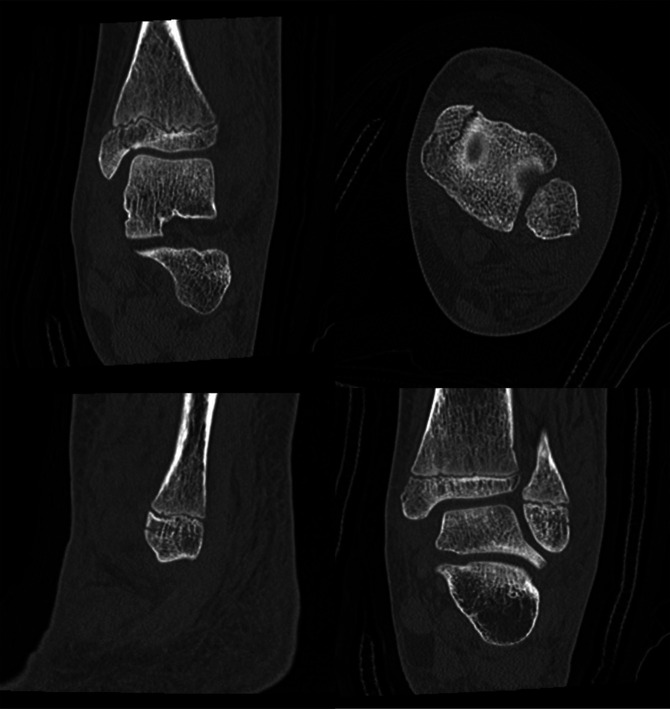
Multiple ankle CT images further demonstrating the nondisplaced fractures of Case 3.
